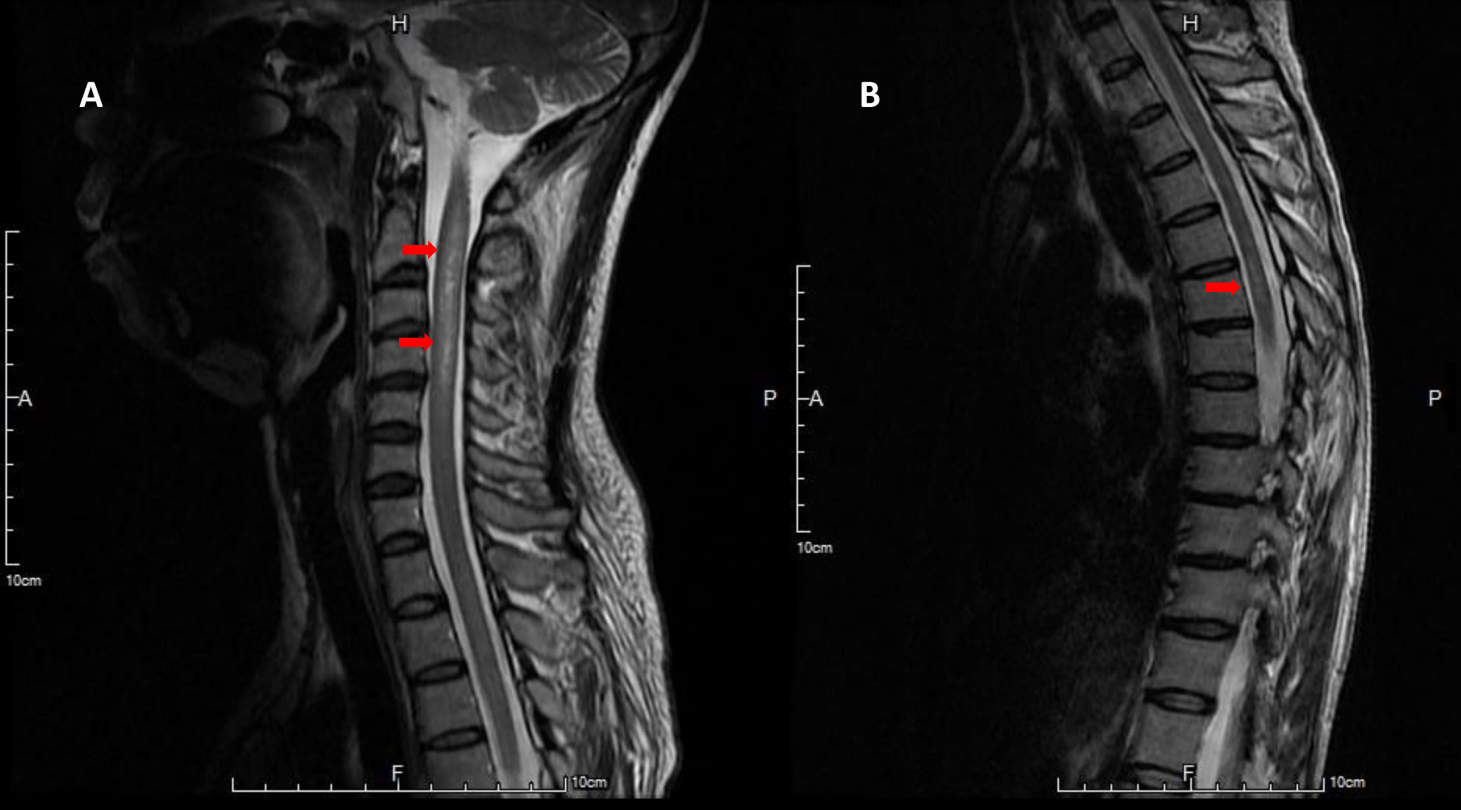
Hyperintense lesions at C2-4 level on T2-weighted image of spine MRI (A). Faint high signal intensities at T5-6 level on T2-weighted image of spine MRI (B).
